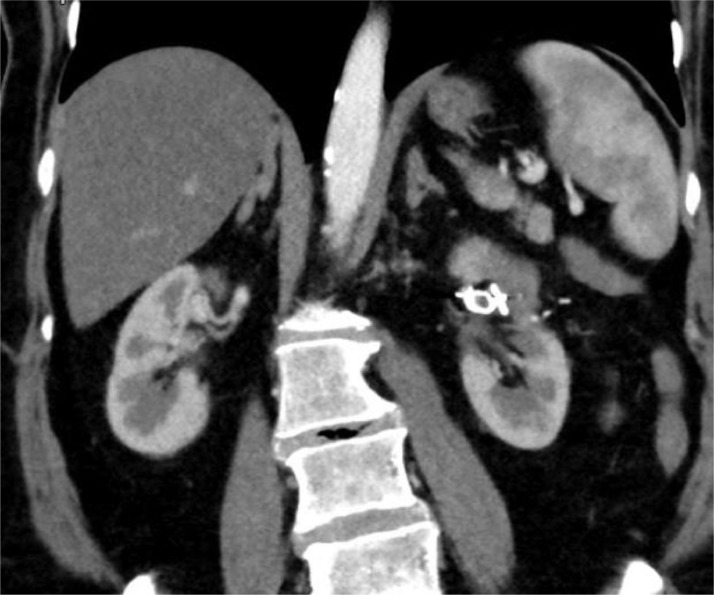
Postembolization CT arterial phase shows absence of the fistula and presence of endovascular coil
